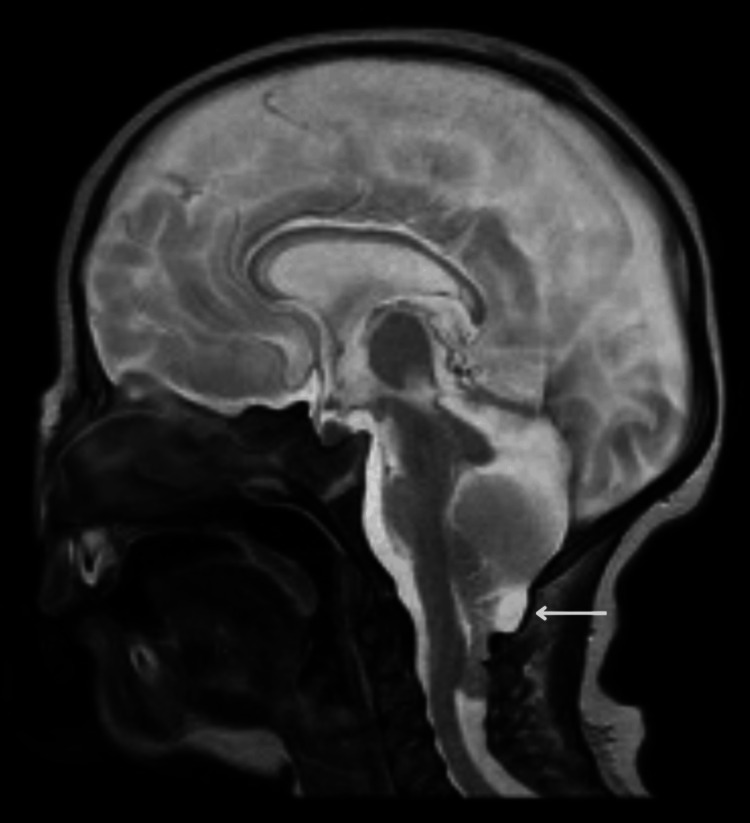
MRI brain midline sagittal T2-weighted MRI brain image that demonstrates a small posterior fossa with evidence of cerebellar tonsillar herniation through the crowded foramen magnum with a collapsed fourth ventricle.
